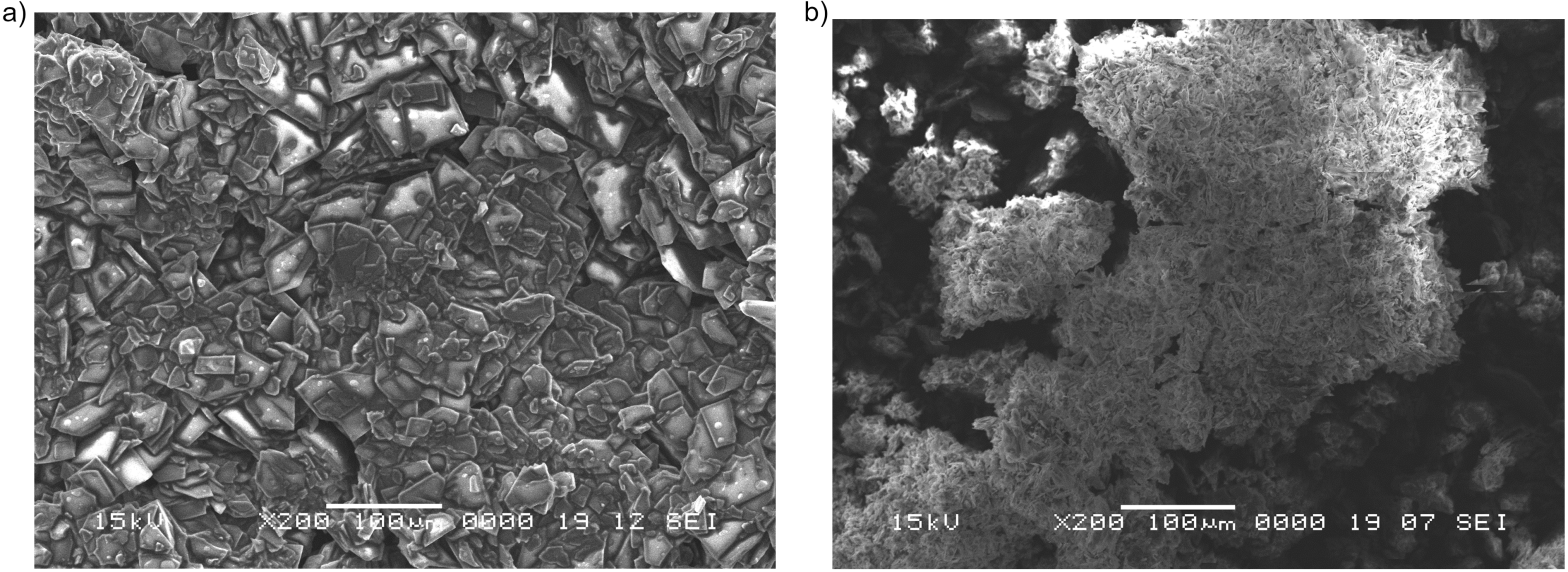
SEM images recorded at 200× for the raw reaction product 2b obtained through a) ultrasound-assisted reaction conditions and b) classical reaction conditions.
